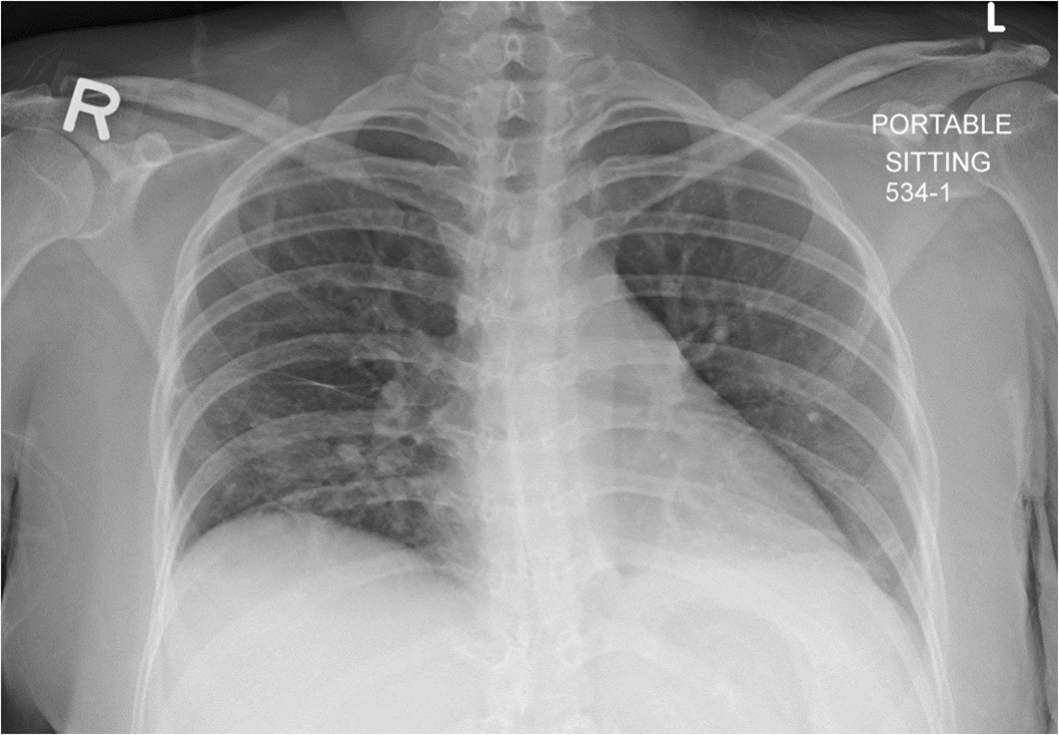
Chest radiograph showing complete resolution of the previously seen bilateral pleural effusion with some bibasilar atelectatic changes.
